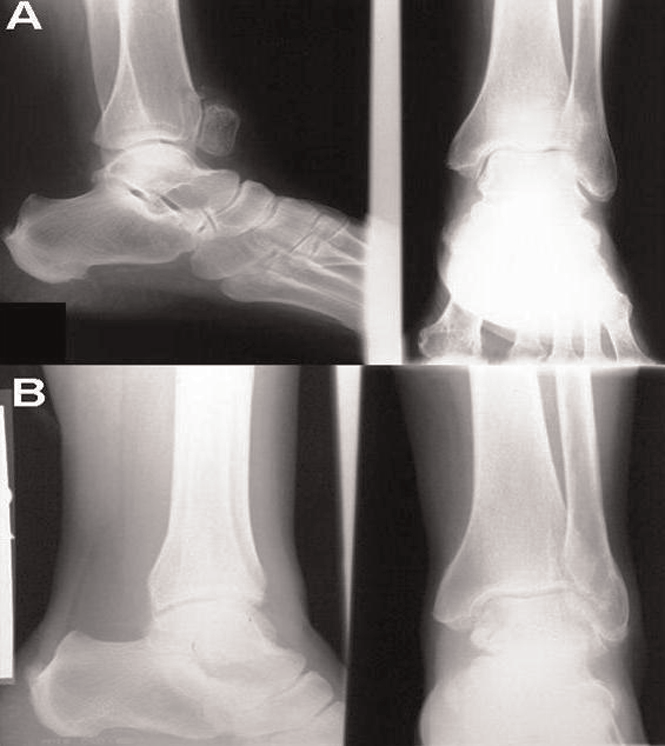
(A) Anteroposterior and lateral X-rays of left leg. (B) Anteroposterior and lateral X-rays of right leg.
